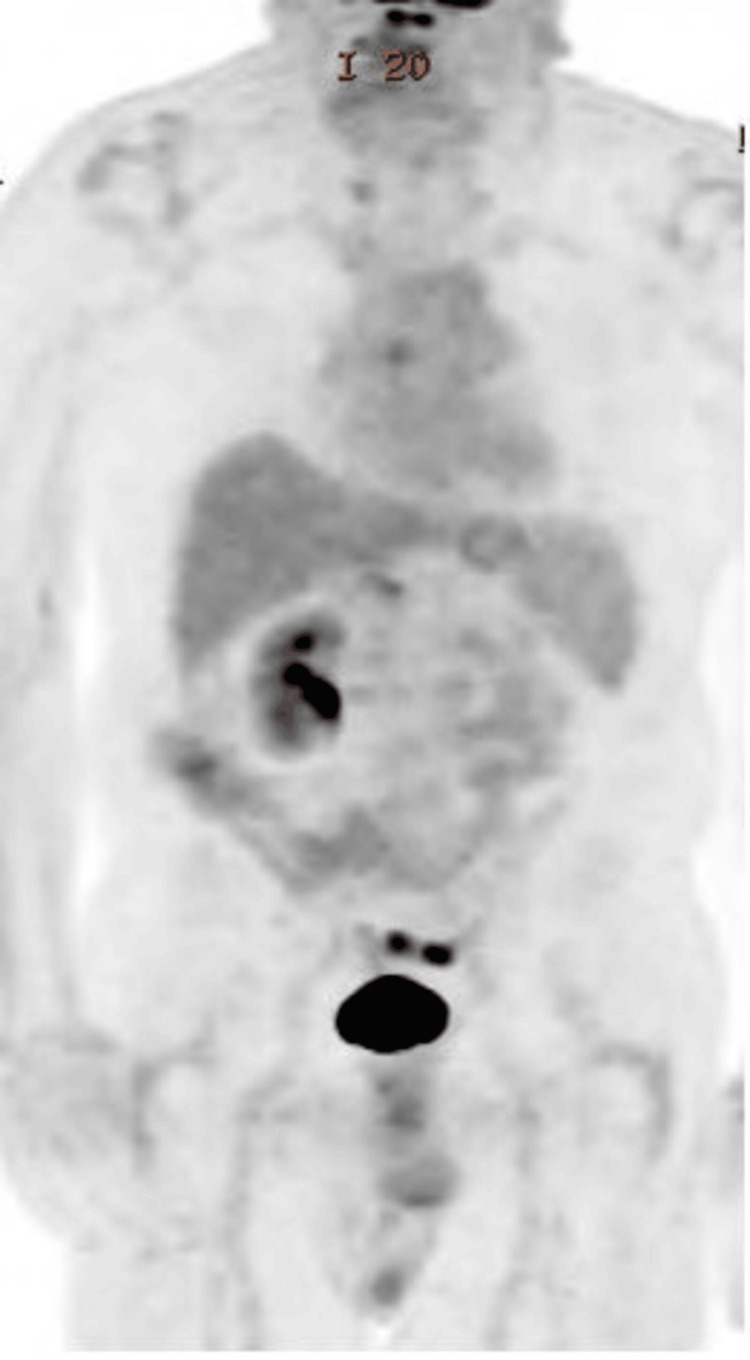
PET scan showing no evidence of FDG-avid disease. FDG: fluorodeoxyglucose.
